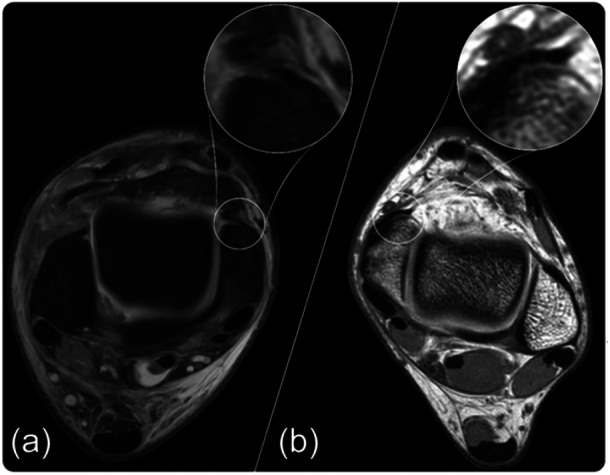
T1 (a) and T2 (b) axial MRI sequence of the ankle joint, showcasing a partial medial sleeve rupture with visible fluid between the anterior portion of the sleeve and medial malleolus.
